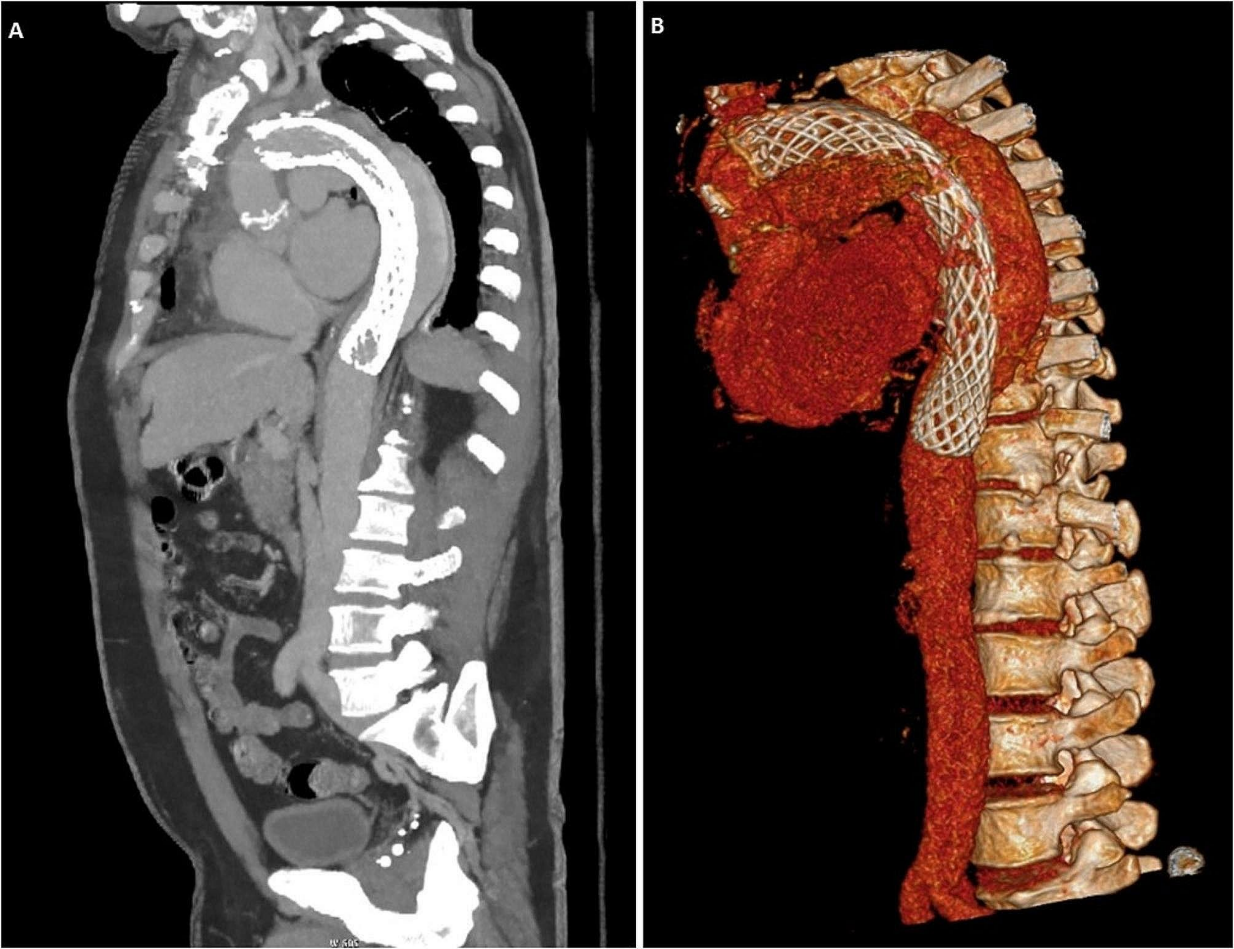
(A) CT aorta post repair showing resolution of the perigraft seroma with no contrast extravasation. (B) 3D reconstruction showing the FET within the AMDS
